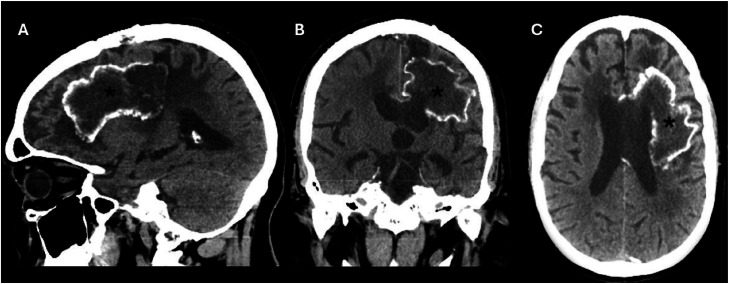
Non-contrast head computed tomography demonstrating sagittal (A), coronal (B), and axial (C) reformatted images. A large lobulated lesion with peripheral calcifications is identified in the anterior aspect of the left centrum semiovale/corona radiata (asterisks), showing central hypoattenuation suggestive of treatment-related changes (coagulative necrosis induced by antiangiogenic therapy). Additional cortical hypoattenuation is noted in the left frontal lobe, consistent with sequelae.
